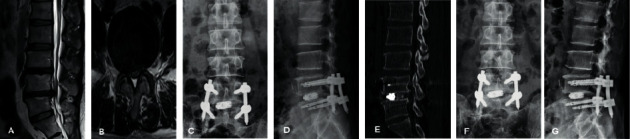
A 65-year-old female patient was diagnosed as lumbar spinal stenosis with degenerative instability and was treated by PE-TLIF. (a, b) Preoperative MRI demonstrated a lumbar spinal stenosis at L4/5. (c, d) A good implantation position was presented through X-ray images taken 7 days after the operation. (e) A standard lumbar fusion was presented via CT scan image at 6 months after the operation. (f, g) X-ray images demonstrated a good implantation position at final follow-up.
